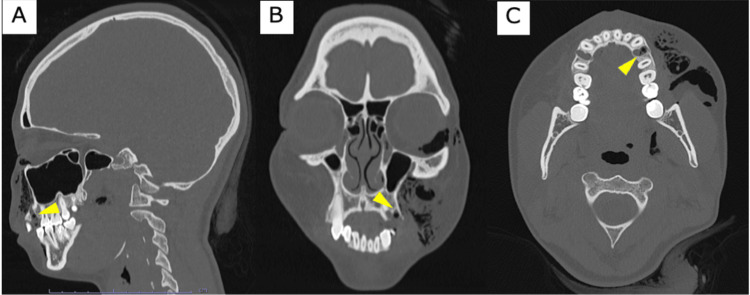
Facial emphysema Computed tomography of the neck and head at the time of emergency department presentation: (A) sagittal, (B) coronal, and (C) axial views. Radiolucent air pockets (dark areas) are visible in the subcutaneous tissues of the left midface, extending into the periorbital region. The likely point of entry is the extraction site of the left maxillary first premolar (indicated by the yellow triangle).
